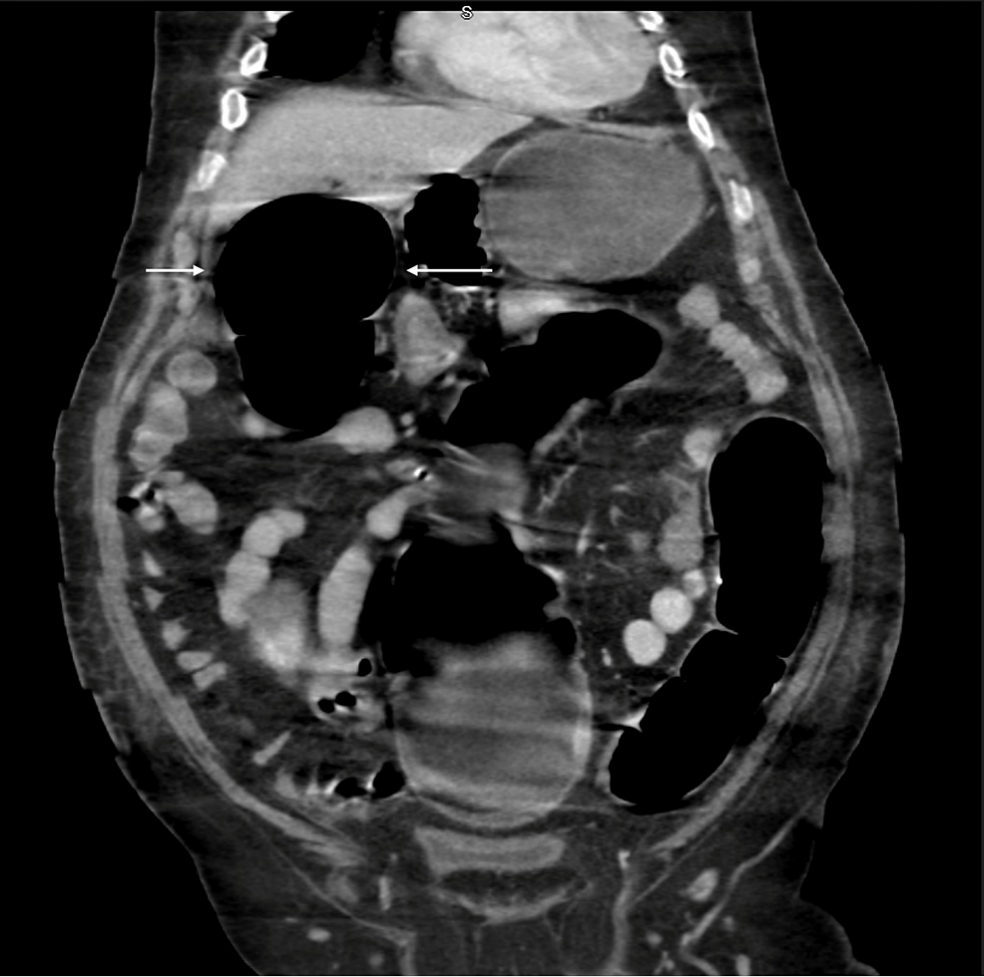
Abdominal CT in coronal view shows severe colonic dilatation (White arrows).
